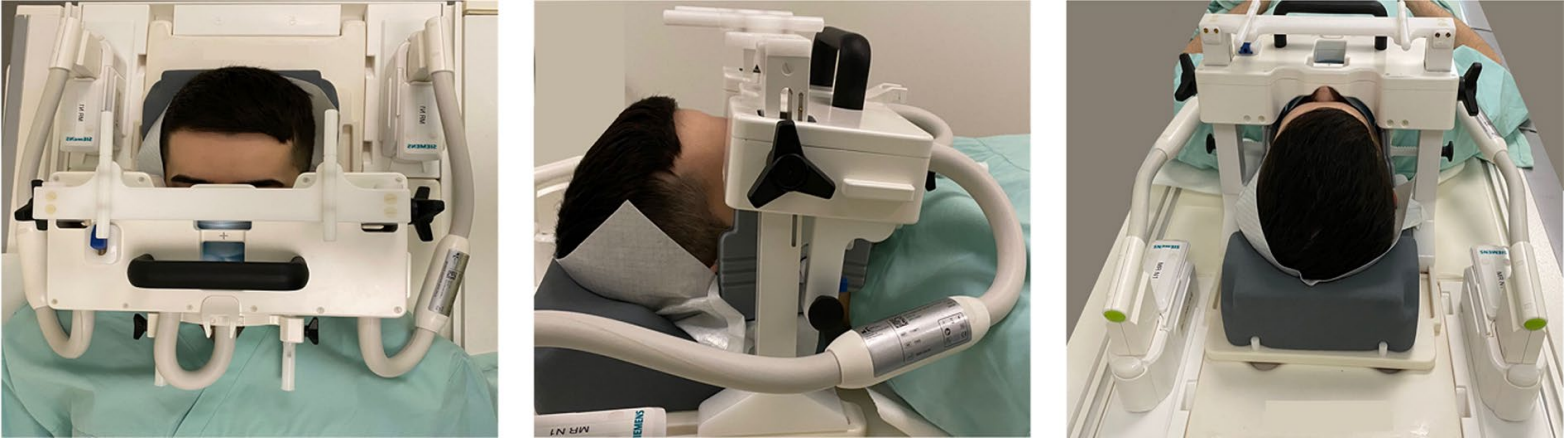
All study participants underwent 3 Tesla MRI (Skyra, release VE11e, Siemens Healthineers, Erlangen, Germany) using a dedicated 15-channels mandibular coil (NORAS MRI products, Hoechberg, Germany). The mandibular coil used in this study has a field of view of 32 × 16 × 16 cm^3^ and is an optimized 14+ 1 receiver coil array and positioning system specifically designed for high-resolution imaging of dental structures in the oral cavity.
